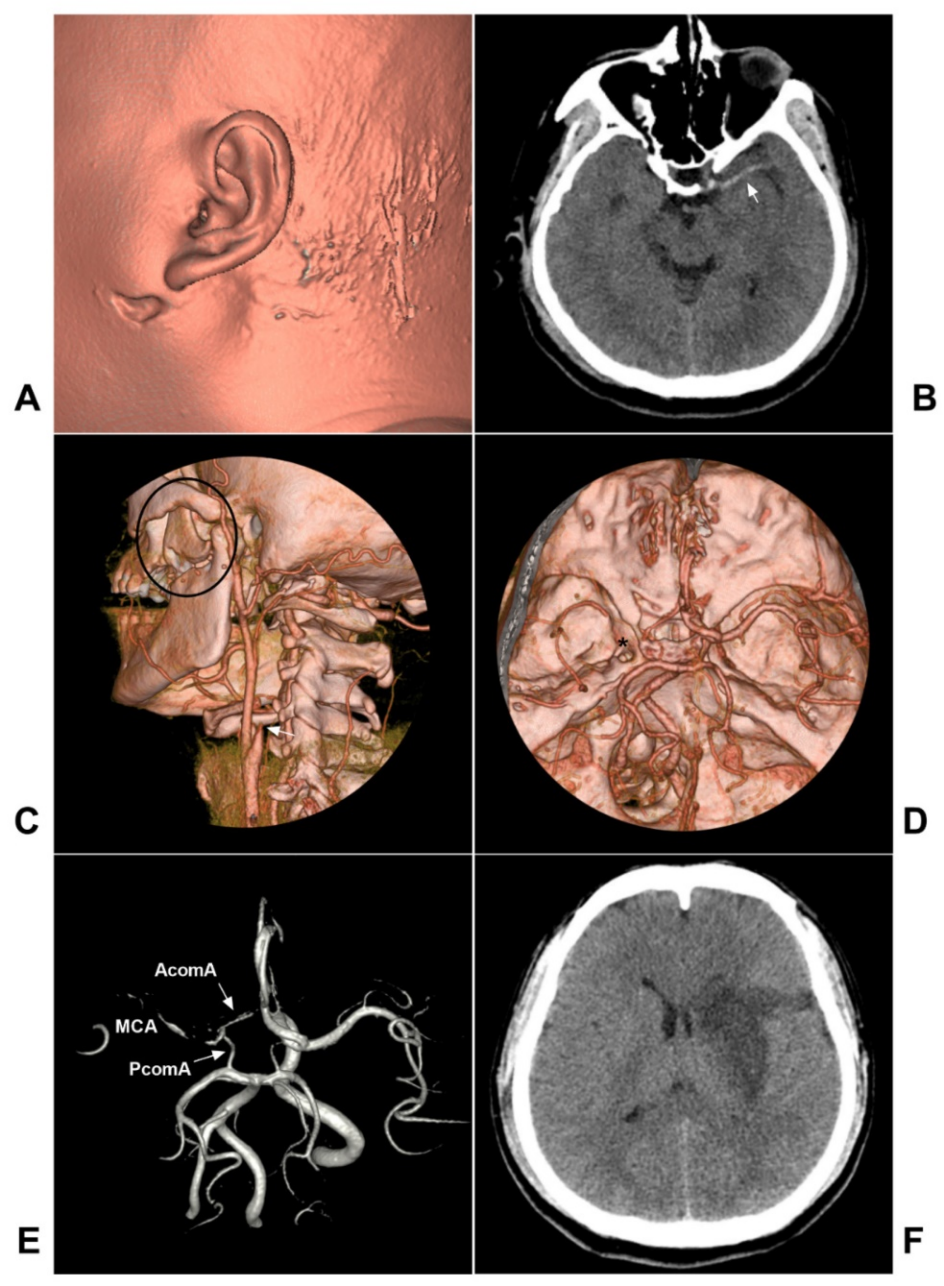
Traumatic extracranial ICA occlusion. A: CTA soft tissue reconstruction shows the left face injury in front of the ear. B: CT showed the left MCA high-density sign (arrow). C: CTA reconstruction showed maxillary bone fracture (black ellipse) and extracranial ICA occlusion (arrow). D: CTA reconstruction showed a thin intracranial MCA (asterisk). E: MRA showed the connections from AcomA and PcomA to MCA. F: Follow-up CT showed infarction of the left basal ganglia region. Abbreviations: AcomA: anterior communicating artery; CT: computed tomography; CTA: CT angiography; ICA: internal carotid artery; MCA: middle cerebral artery; MRA: magnetic resonance angiography; PcomA: posterior communicating artery
